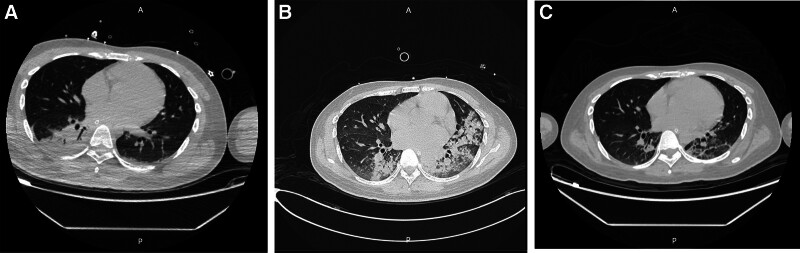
(A) Computed tomography (CT) of the chest on admission revealed bilateral pneumonia, incomplete expansion of the right lower lobe, and a small amount of pleural effusion on both sides. (B) Chest CT on the second week of admission showed that bilateral pneumonia had progressed compared with the previous period. (C) Chest CT on the 5th week of admission showed that bilateral pneumonia had absorbed compared with the previous period.
